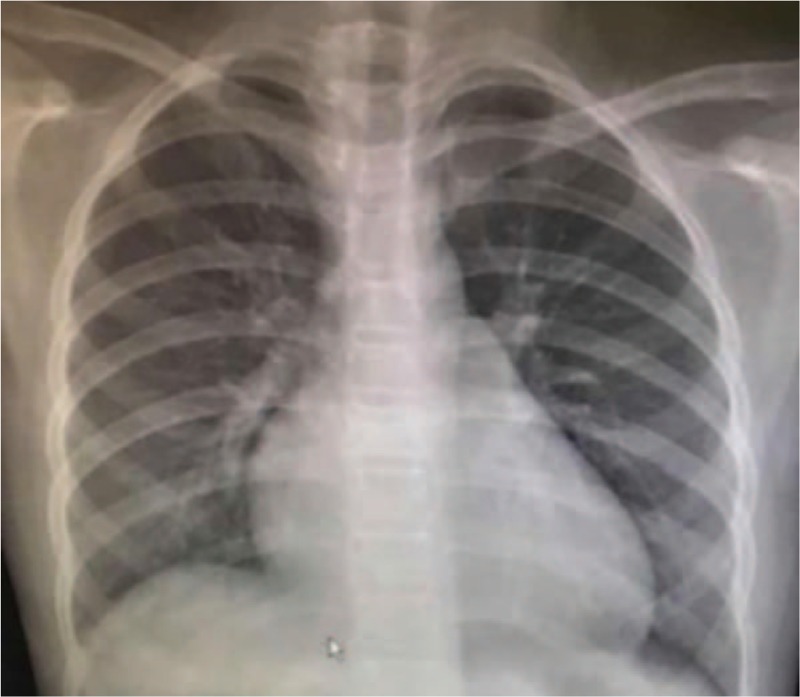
Chest radiography found heart shadow enlargement, and the cardiothoracic ratio was 0.62.
